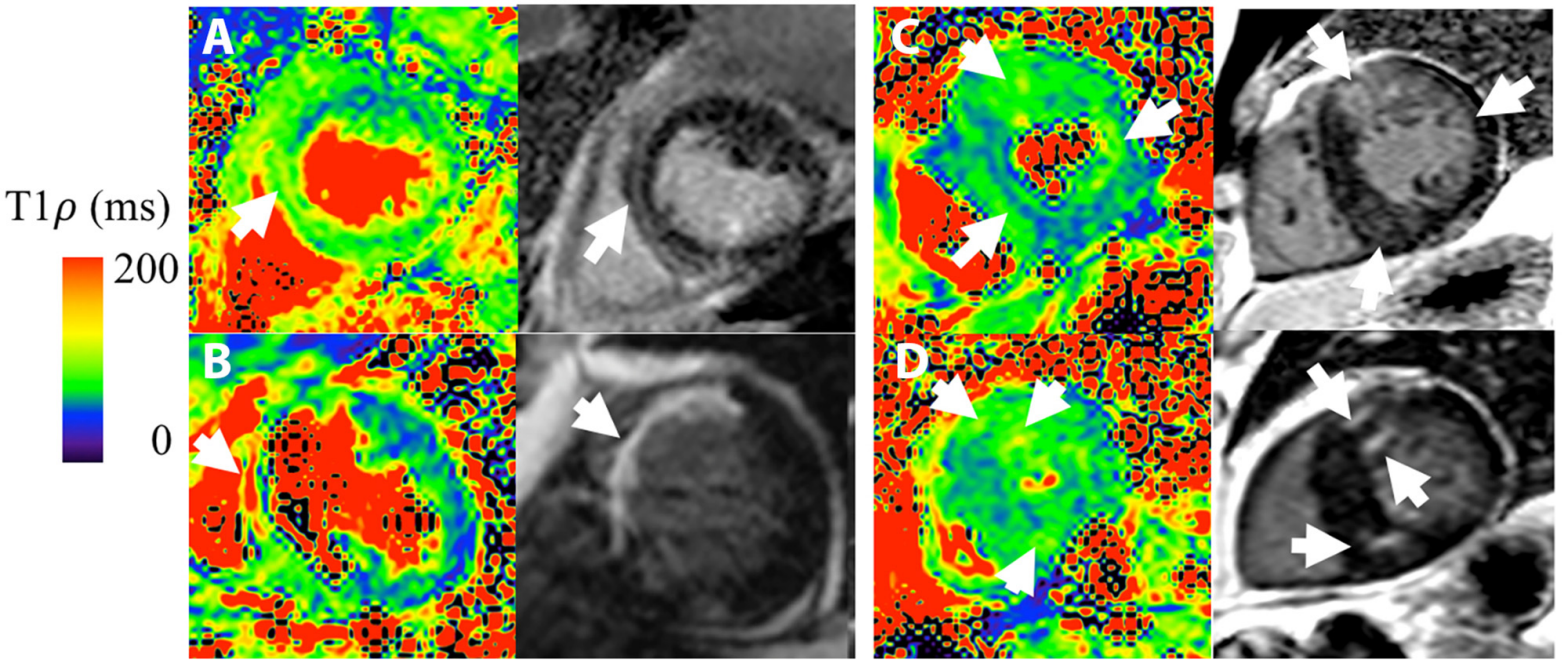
T_1ρ_ and LGE MRI in patients. Subjects with A, PVCs, B, chronic myocardial infarction, and C-D, HCM. Spatial correspondence between increased T_1ρ_ and LGE MRI is indicated with white arrows. LGE images were obtained in end-diastole and T_1ρ_ in end-systole.
